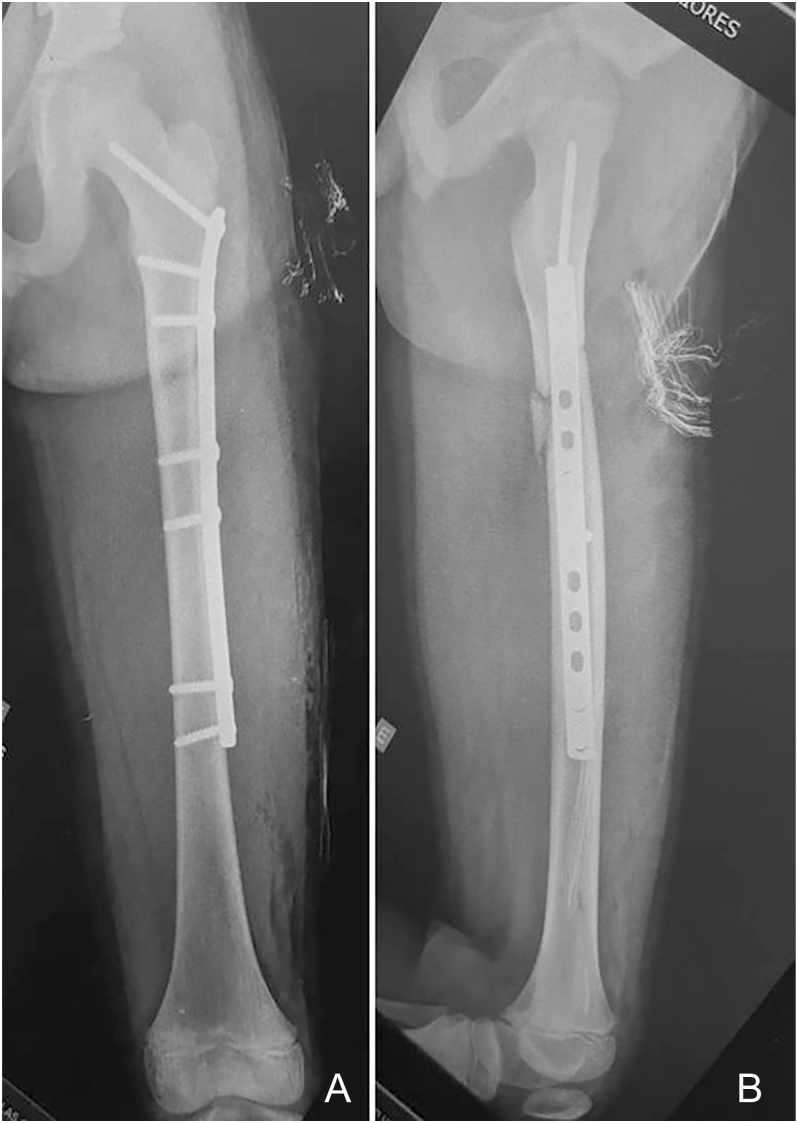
Radiographs showing screws arranged in the dispersed pattern, also known as near–near, far–far pattern: anteroposterior view (A); lateral view (B).
